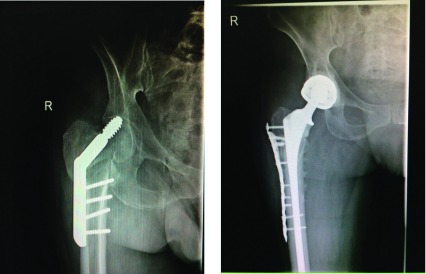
Radiograph of patient with failed DHS revised to dual mobility THR. Locking plate used to stabilize the trochanteric fracture.
